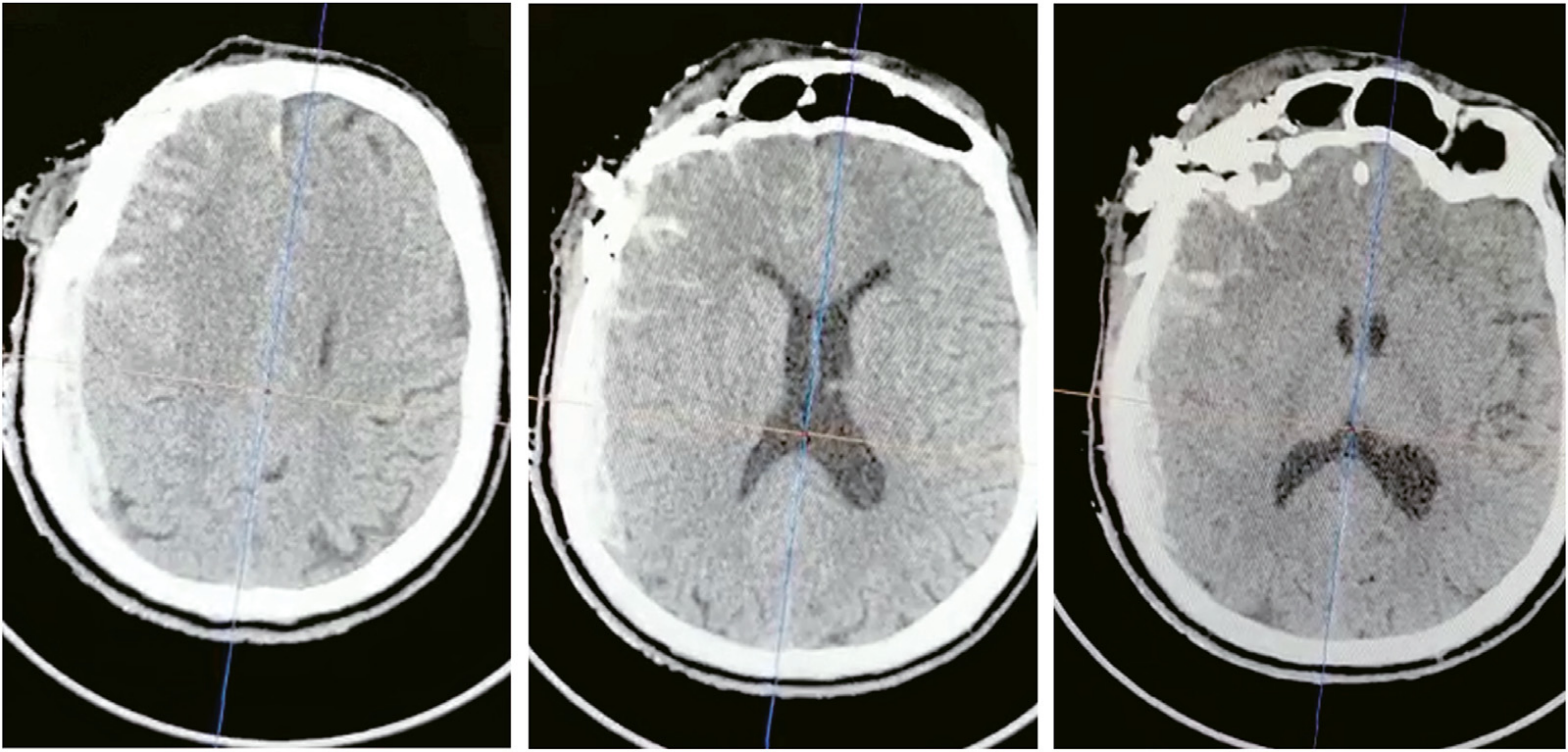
Preoperative axial CT showing right-sided subdural hematoma, effaced basal cisterns and midline shift.
